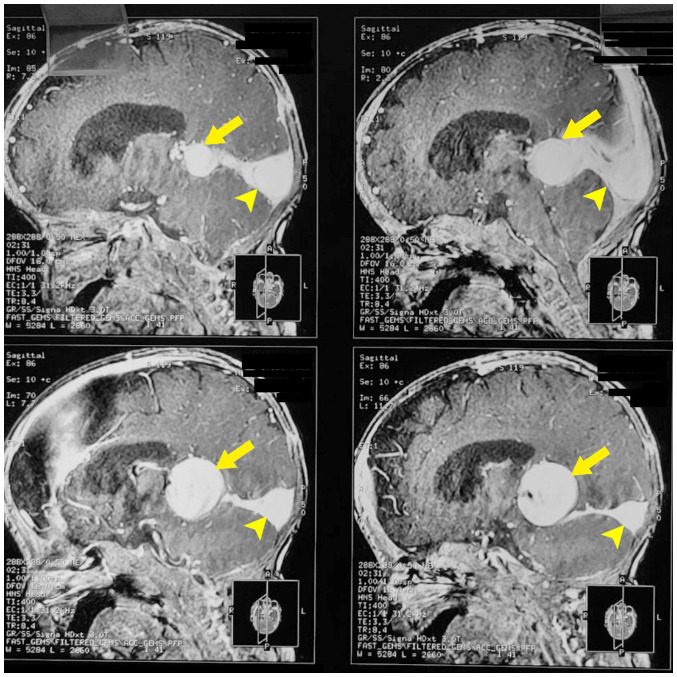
Sagittal T2-weighted magnetic resonance imaging. The dilated galenic vein (yellow arrow), also known as the median vein of prosencephalon, is located midline in the cistern of the velum interpositum and drains into the superior sagittal sinus (yellow arrowhead).
